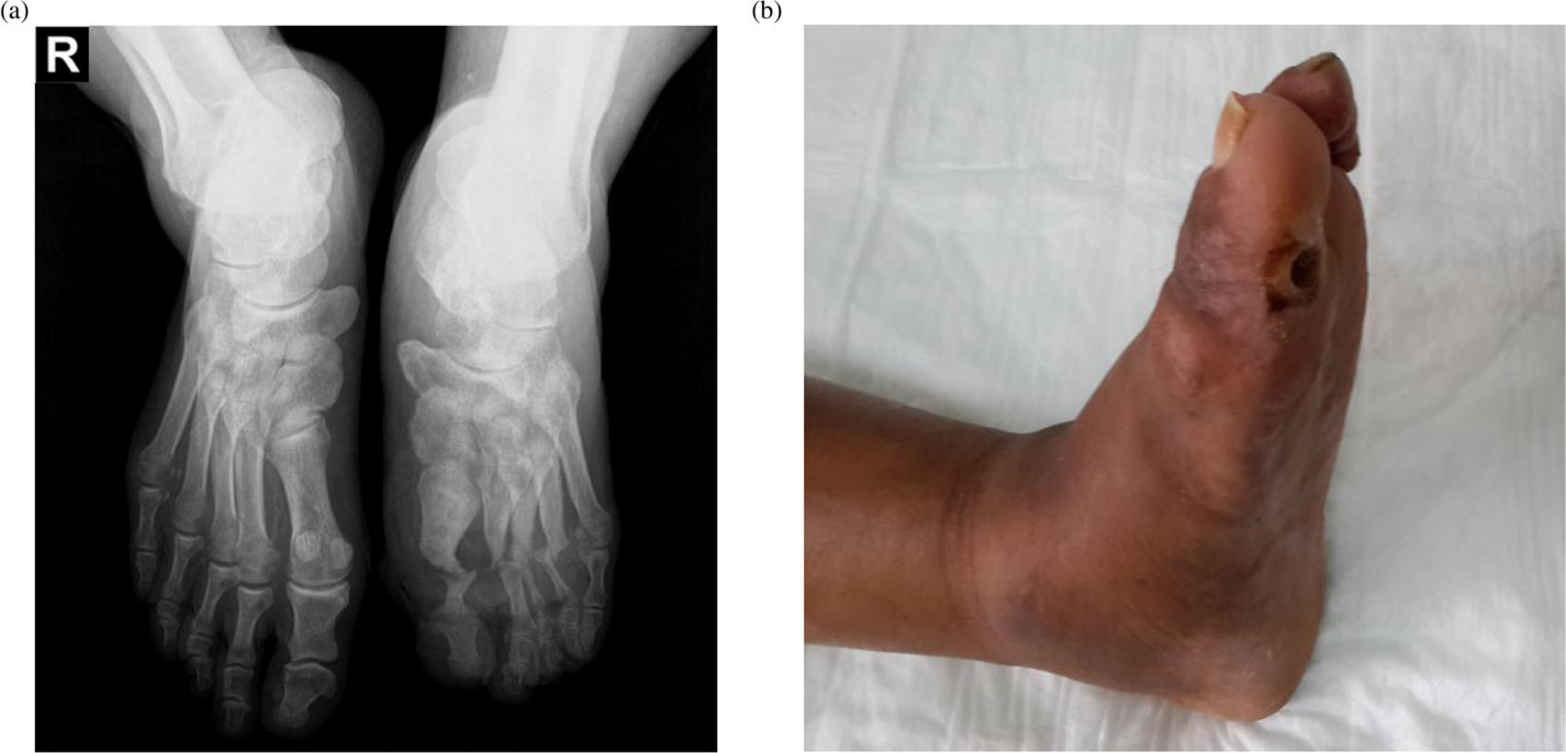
(A) Plain radiographs with the left foot showing typical bony changes in Charcot neuro-osteoarthropathy (bone destruction, joint fragmentation, and dislocation). (B) Photograph of the same patient's left foot with great toe and midfoot deformities, including collapsed arch.
